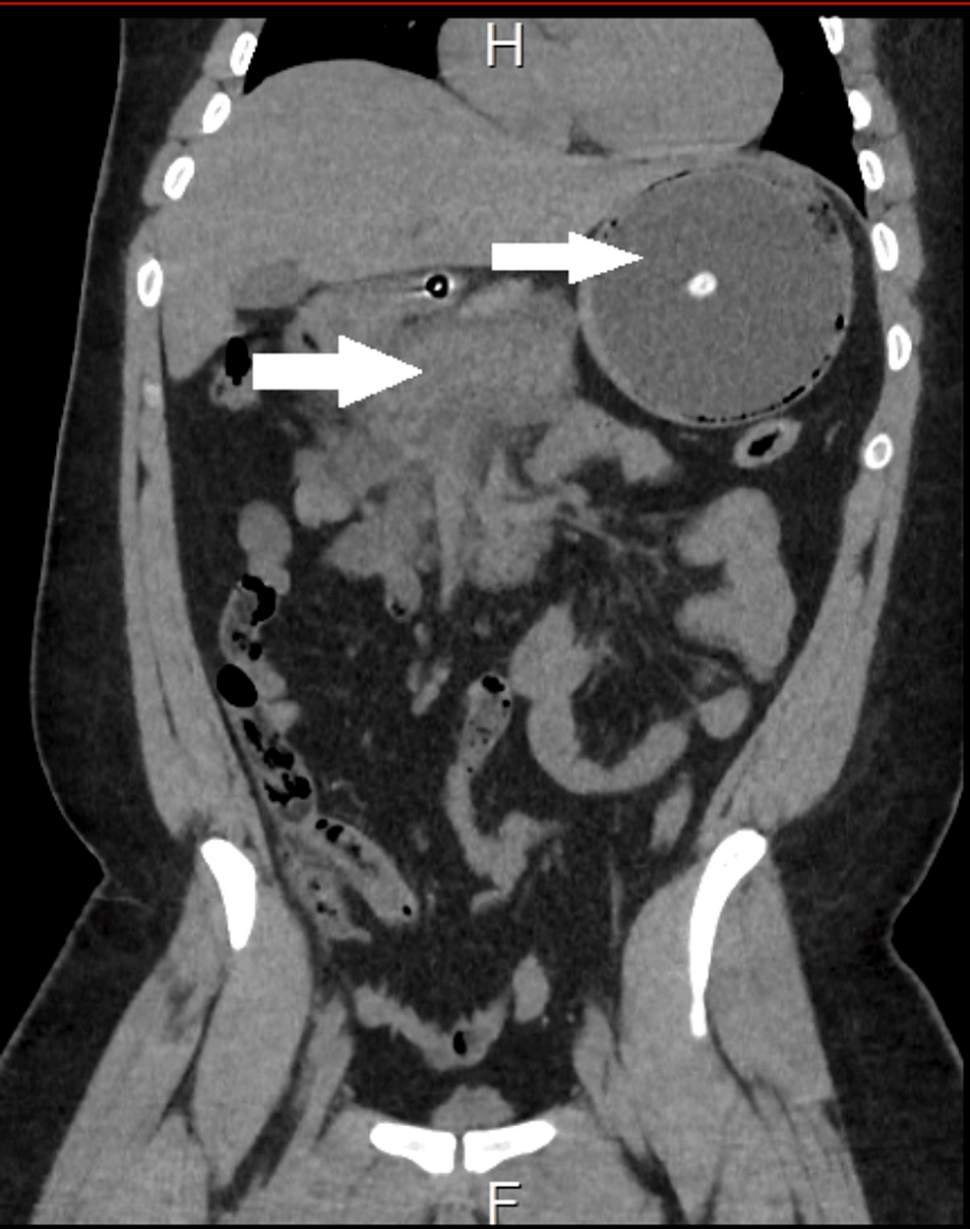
CT scan of the abdomen showing an intragastric balloon with acute pancreatitis and peripancreatic fluid collection CT: computed tomography
